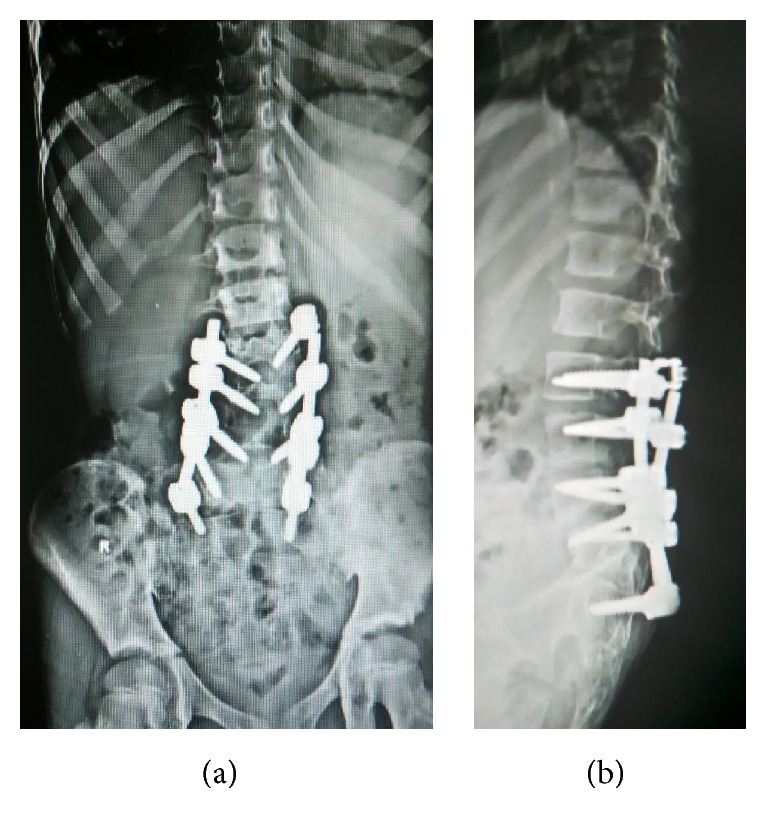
A-P and lateral radiograph of lumbosacral spine after 3-year follow-up.
